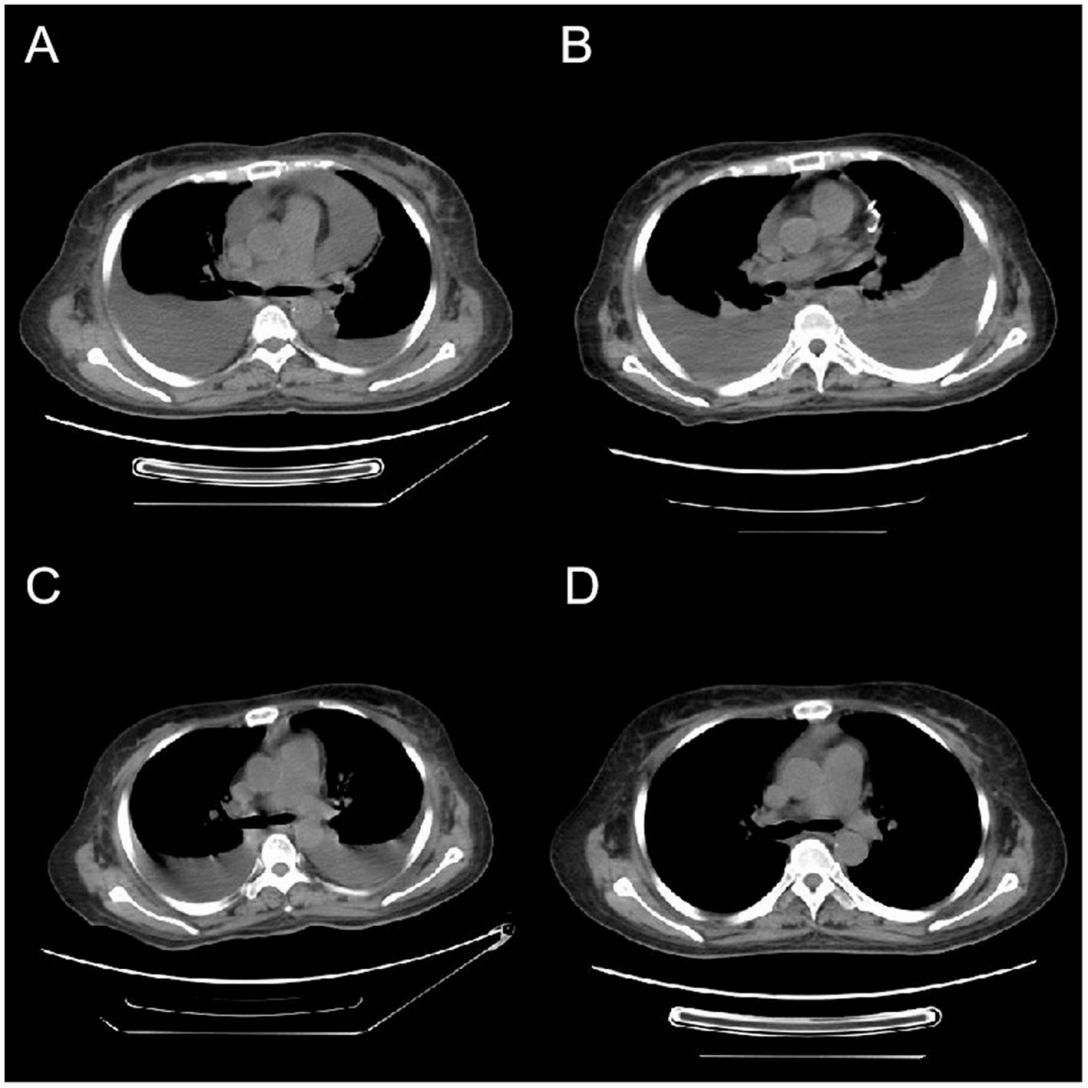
Variations in CT imaging throughout the patient's treatment regimen. (A) The initial CT scan upon admission, revealing the presence of bilateral pleural effusions and a pericardial effusion. Four weeks post-admission, (B) a CT scan that exhibits an augmentation in pleural effusions from baseline, along with the presence of a visible pericardial drainage catheter. (C) A CT scan conducted 3 days following bevacizumab administration, demonstrating a notable decrease in pleural effusions. One week subsequent to the completion of bevacizumab therapy, (D) a CT scan indicating the full resolution of pleural effusions.
